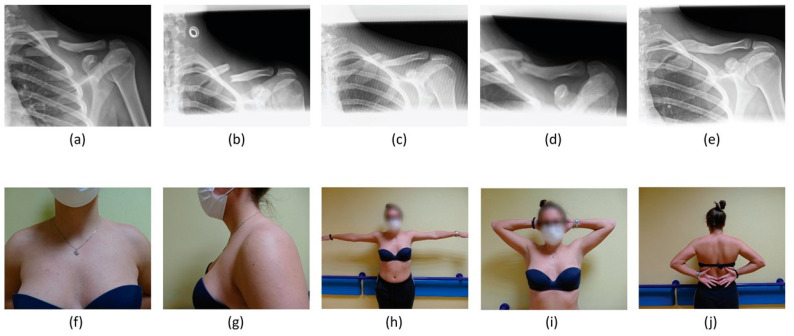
A 24-year-old female patient treated conservatively for an AO A3 left ADMCF. Clavicle radiographic images (a) at the time of patient presentation in ER, (b) at immediate post-reduction radiographic control using a F8-B, (c) at 30-day follow up, (d) at 6-month follow up and (e) at last follow up of 31 months. Last follow-up clinical images showing (f,g) no mass in the supraclavicular fossa and good range of movement in abduction (h), extra-rotation (i) and intra-rotation (j).
